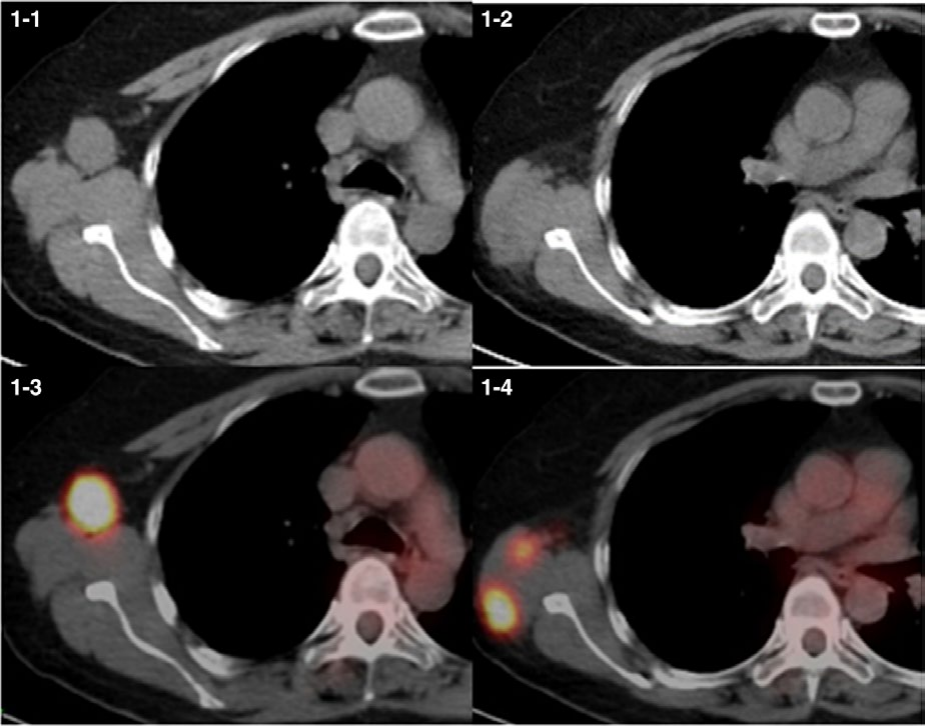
Thoracic CT and FDG‐PET images 26 months after surgery There are metastatic tumors (2.5 cm in diameter) in the right latissimus dorsi muscle (1‐1) and (2 cm in diameter) in the armpit (1‐2). FDG‐PET scan shows uptake in the same areas (1‐3, 1‐4)
